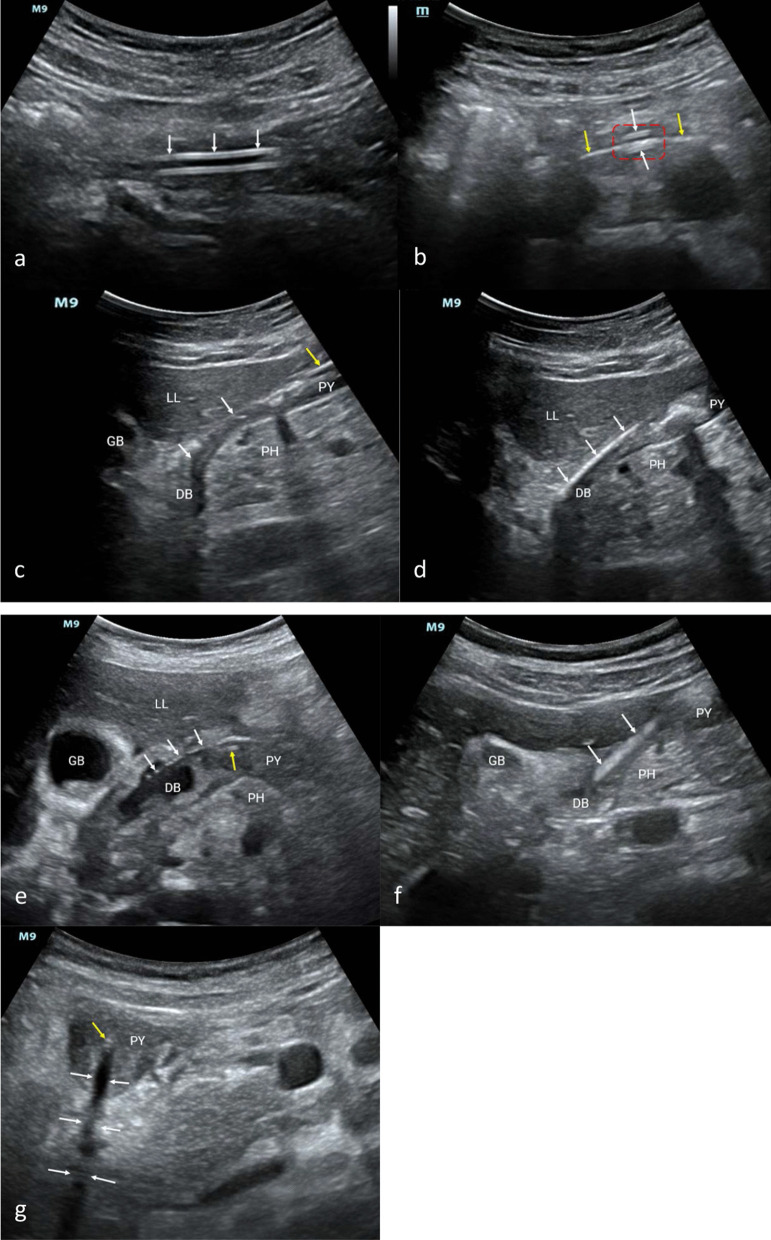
Acoustic signs of the nasointestinal tube (NIT) on ultrasound. a Double-track sign: white arrows; b Five lines sign: red dotted box; Guidewire: yellow arrows; Wall of the NIT: white arrows; c Bar shadow sign: white arrows; NIT: yellow arrow; d: Bright band sign: white arrows; e Gas bead-like sign: white arrows; NIT: yellow arrow; f: Dynamic water flow sign: white arrows; g: Short-axis acoustic shadow sign: white arrows. NIT: yellow arrow. DB, duodenal bulb; GB, gallbladder; LL, left liver; PH, pancreatic head; PY, pylorus
